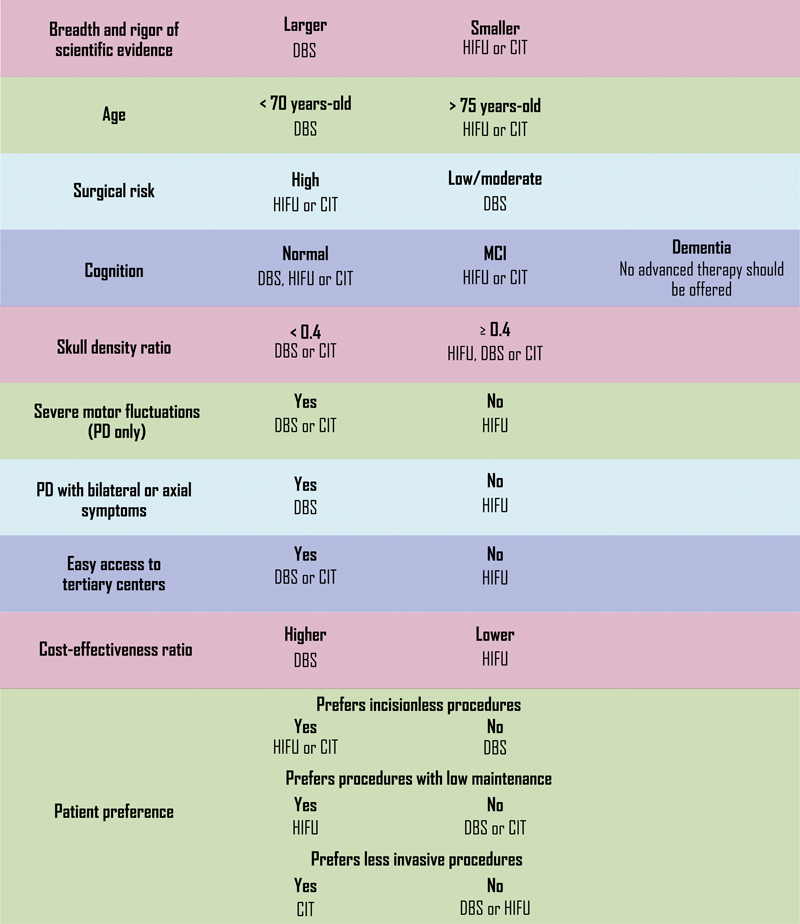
Abbreviations: CIT, continuous infusion therapy; DBS, deep brain stimulation; HIFU, high-frequency focused ultrasound; MCI, mild cognitive impairment.
